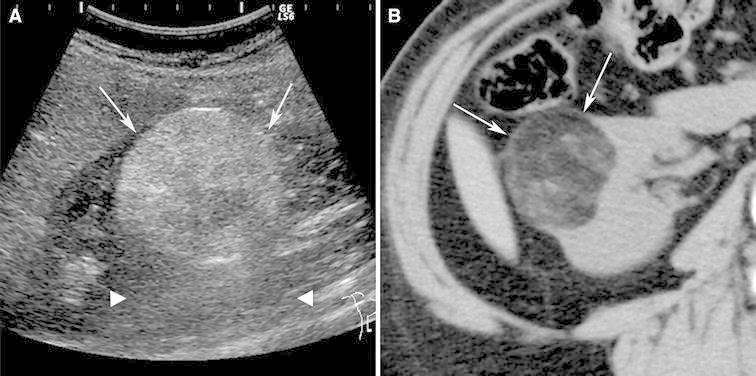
Angiomyolipoma, classic type, in a 61-year-old woman. Ultrasound (A) shows a 4.2-cm right renal mass (*arrows*) that is markedly hyperechoic relative to renal parenchyma and accompanied by acoustic shadowing (*arrow heads*). Transverse, unenhanced CT image (5-mm sections) shows a right renal mass (*arrows*) with fat attenuation (−60 HU).
